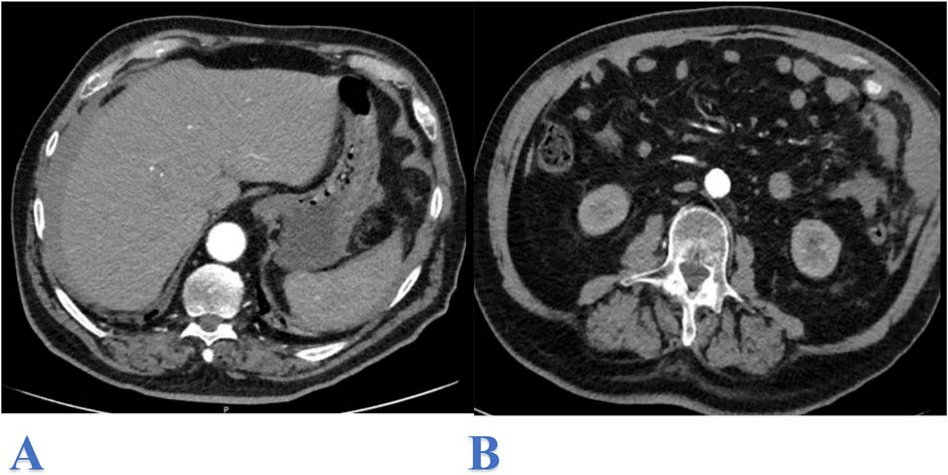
Axial CT abdomen image (arterial phase) showing: bilateral subphrenic hemoperitoneum (A) and contrast extravasation from the upper left lateral omentum (B).
